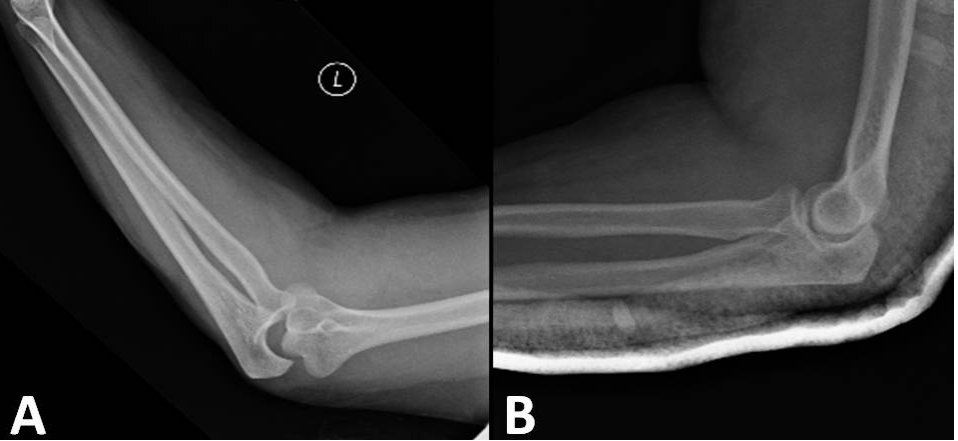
Same case as in figure 3. X-rays before (panel A) and after (panel B) closed reduction of a left elbow dislocation.
